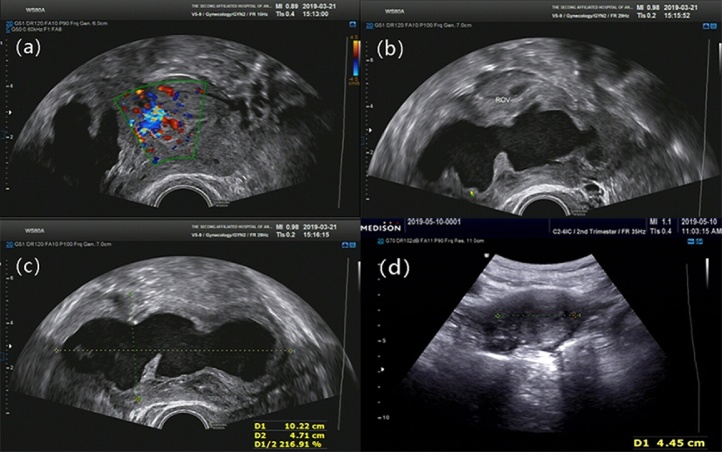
Ultrasound images. (a) Blood flow signals around the cyst. (b) (c) Cyst on the right side of the uterus. (d) One month after surgery.
